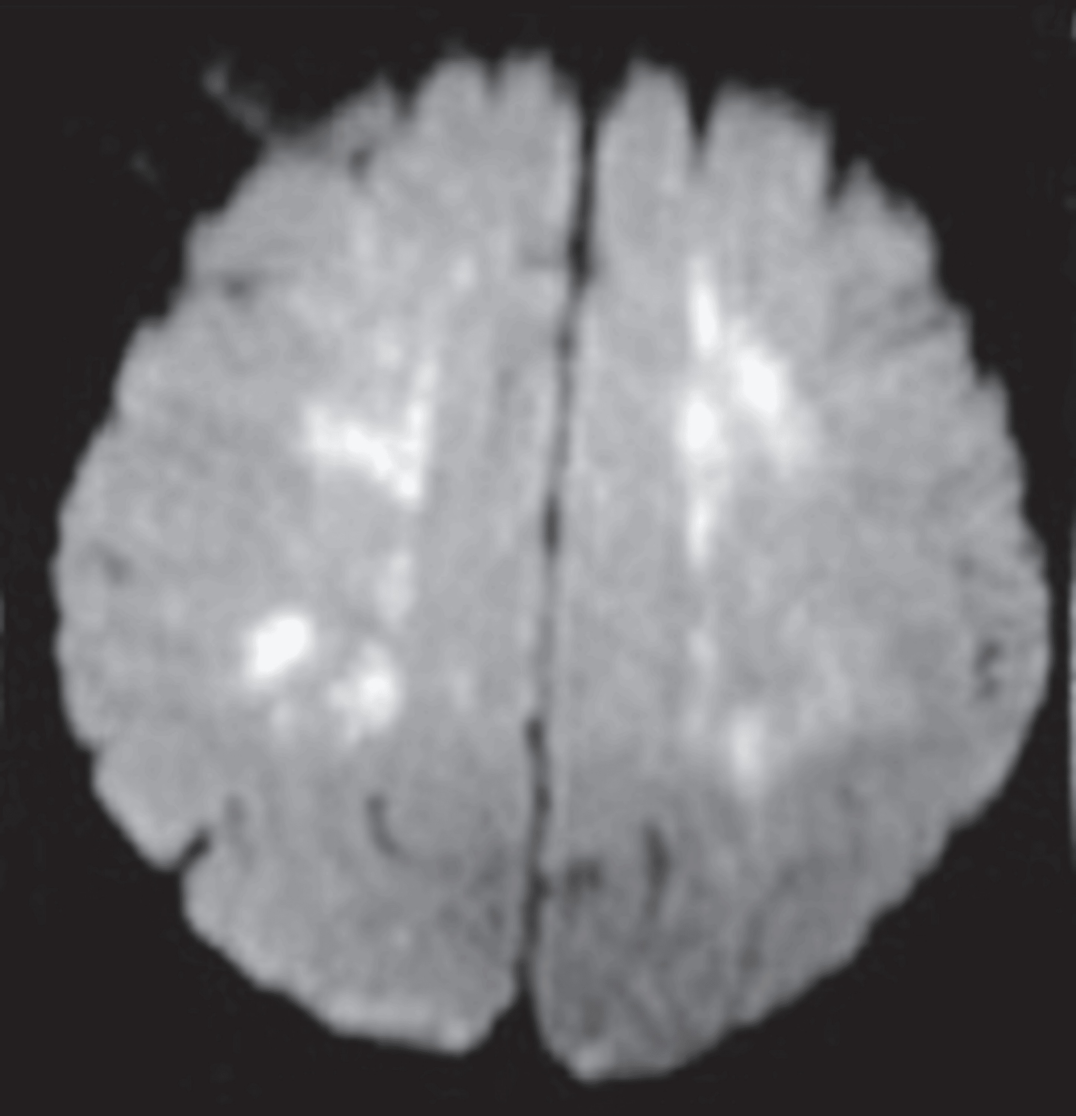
Starfield pattern of MRI brain in FES. This figure was obtained from Dr. Nissar Shaikh’s image collection; the patient's informed consent was obtained. FES: fat embolism syndrome
